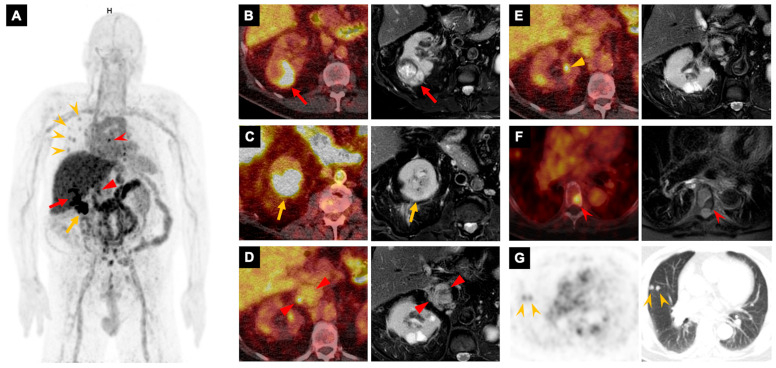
A 78-year-old man undergoing surveillance for Bosniak IIF left renal cysts was found to have an enlarging right interpolar renal mass. Shown are the ^89^Zr-girentuximab PET whole-body maximum intensity projection image (A), axial PET/CT fused images (left in (B–F)), axial T2-weighted fat-suppressed MRI (right in (B–F)), an axial PET image (left in (G)), and the corresponding lung-window CT image (right in (G)). The right interpolar mass demonstrates heterogeneously intense uptake (red arrows in (A,B)). Importantly, ^89^Zr-girentuximab PET reveals a second intensely avid right lower pole renal mass (yellow arrows in (A,C)) that is occult on MRI, where it exhibits only subtle T2 hypointensity. Additional foci of intense uptake are present within the expanded inferior vena cava (red triangles in (A,D)) and a right renal vein branch (yellow triangles in (E)), indicating tumor thrombus; the venous branch involvement is not conspicuous on MRI. A lesion in the T6 vertebral body (red arrowheads in (A,F)) and multiple pulmonary nodules (yellow arrowheads in (A,G)) also demonstrate uptake, consistent with metastatic disease. Biopsy of the right interpolar mass confirmed clear-cell renal cell carcinoma.
